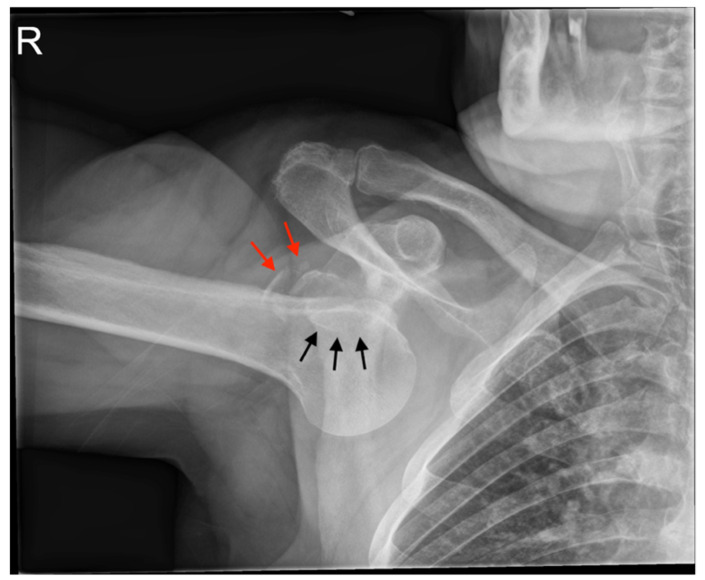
Plain radiography of the right shoulder with inferior dislocation: the arm is fixed in abduction under the inferior glenoid rim (black arrows), the greater tuberosity is fractured (red arrows).
